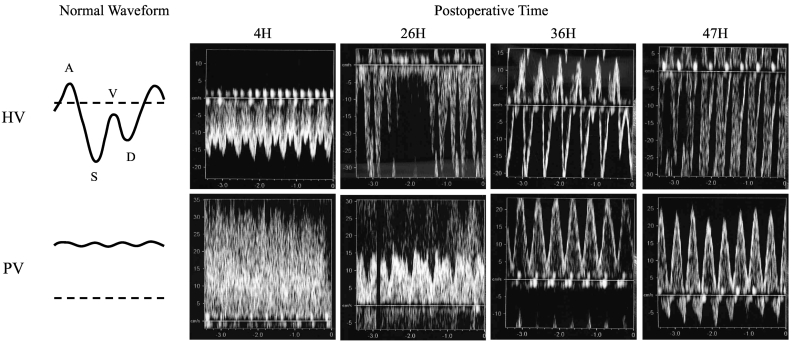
Changes observed in abdominal Doppler US; Doppler US of the right HV and the right PV in the right lobe graft. Abbreviations: H, hours; HV, hepatic vein; PV, portal vein; US, ultrasonography.
